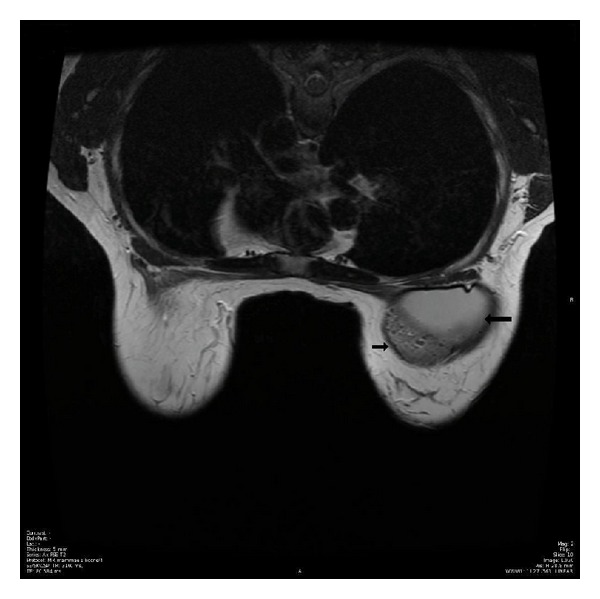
MRI scan showing the gossypiboma (small arrow) as a well-defined area dotted with silicone-like material above the breast implant (large arrow) on the right side, corresponding to the area where the patient felt a painful lump. The implant in the left breast is not visible in this slide.
